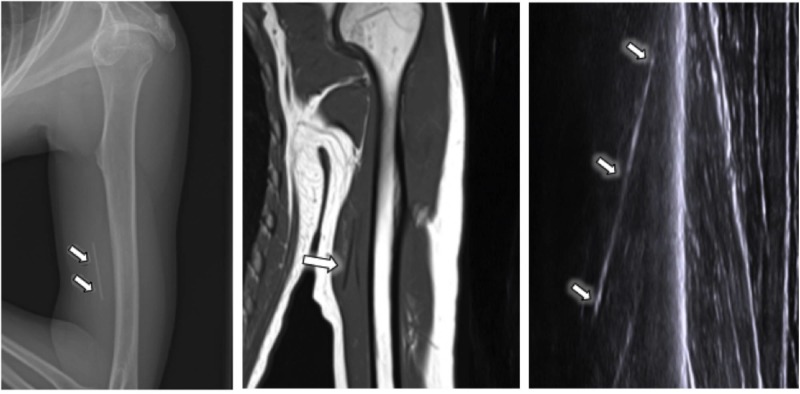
Radiograph, MRI, and an ultrasound image of the left upper extremity A radiograph, MRI, and an ultrasound image of the left upper extremity show an implantable birth control device (arrows) inappropriately located within the triceps musculature. Note the geographic appearance of the 4 cm device completely surrounded by muscle tissue, the lack of acoustic shadowing, and the similarity to other fascial planes on ultrasound. MRI: magnetic resonance imaging
